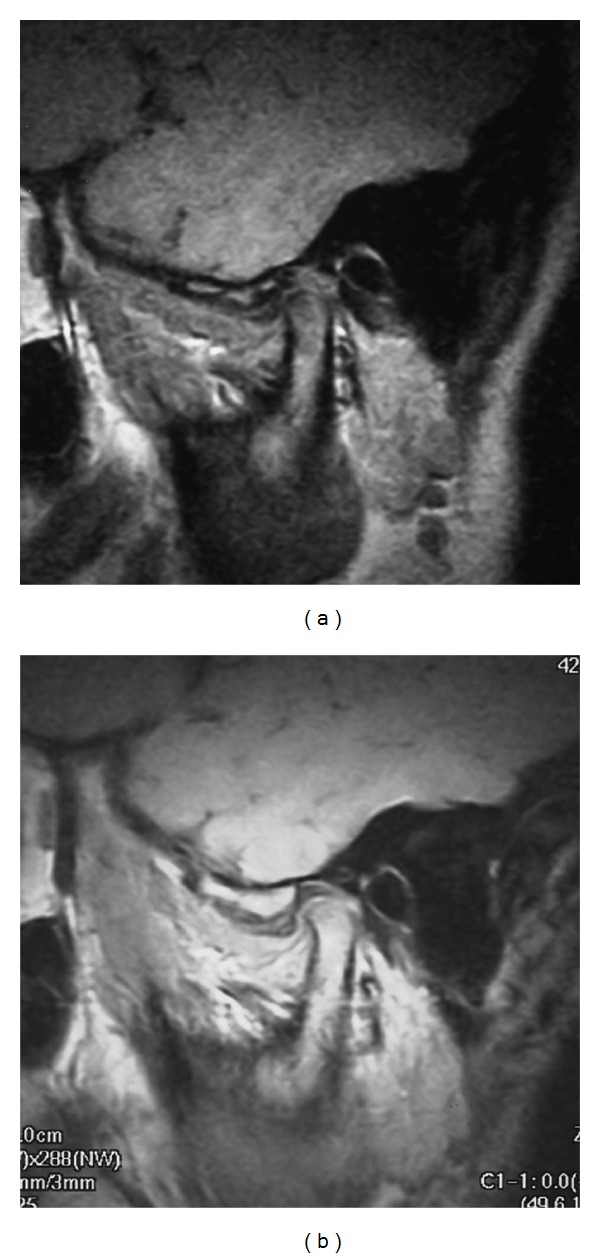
Differences in the MRI signal within the articular eminences and mandibular condyles. (a) Morphology of the articular eminences and glenoid fossa of TMJ on MRI is in conformity with the outline of the mandibular condyles on fast spin-echo intermediate-weighted images of a healthy 10-year-old boy at the first examination. MRI signal of the articular eminence was yellow marrow, but that of mandibular condyle was red. The imaging parameters used in the MR sequences included 1050 ms TR, 15 ms effective echo time, flip angle of 90°, a 15 × 15 cm field of view, a matrix of 160 × 288 pixels, 17 mm slab thickness, and 3 mm section thickness. (b) Morphology of the articular eminences and glenoid fossa of TMJ on MRI is in conformity with the outline of the mandibular condyles on the second examination three years after the first examination in the same subject as Figure 4(a). MRI signal of the articular eminence was of yellow marrow, and that of the mandibular condyle was also yellow marrow. The imaging parameters used in the MR sequences included 1050 ms TR, 15 ms effective echo time, flip angle of 90°, a 15 × 15 cm field of view, a matrix of 160 × 288 pixels, 17 mm slab thickness, and 3 mm section thickness.
